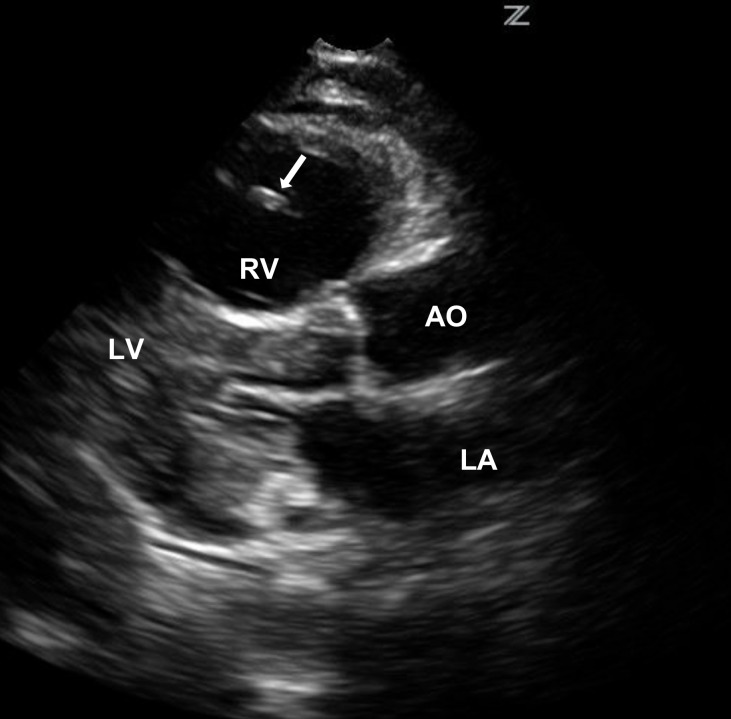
Parasternal long view of the heart with the free-floating clot (arrow) visualized in the dilated right ventricle (RV). *LA*, left atrium; *LV*, left ventricle; *AO*, aortic outflow
